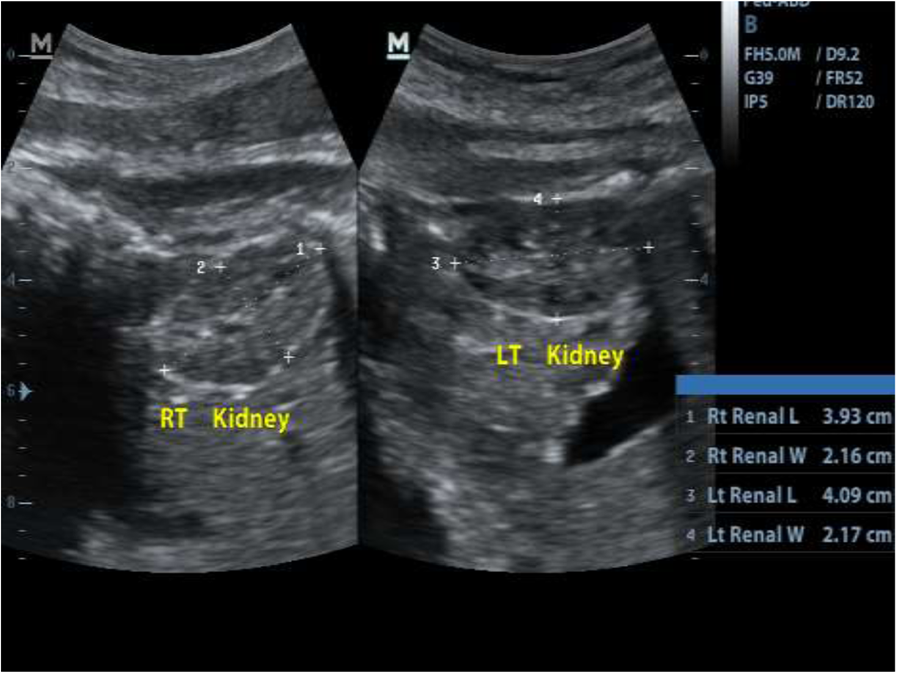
Sonogram of fetal kidney lengths measurement at 35 weeks of gestation
